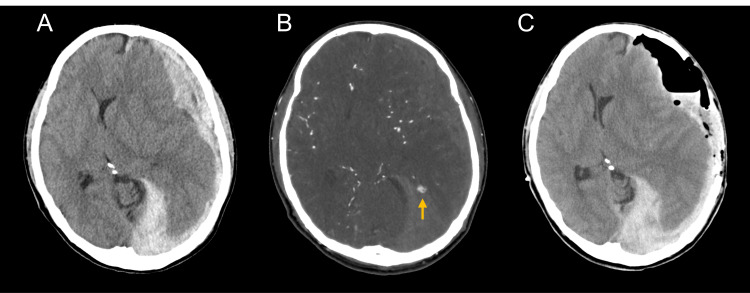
An acute subdural hematoma and an aneurysm at the quadrigeminal segment (P3 segment)/cortical segment (P4 segment) of the left posterior cerebral artery Initial emergent computed tomography (CT) scan showed an acute subdural hematoma covering a large area of the left cerebral hemisphere and uncal herniation without subarachnoid hemorrhage (A). CT angiography revealed an irregularly shaped aneurysm at the quadrigeminal segment (P3 segment)/cortical segment (P4 segment) of the left posterior cerebral artery (arrow (B)). The CT scan performed immediately after the decompressive craniectomy showed that most of the frontotemporal hematoma was removed (C).
